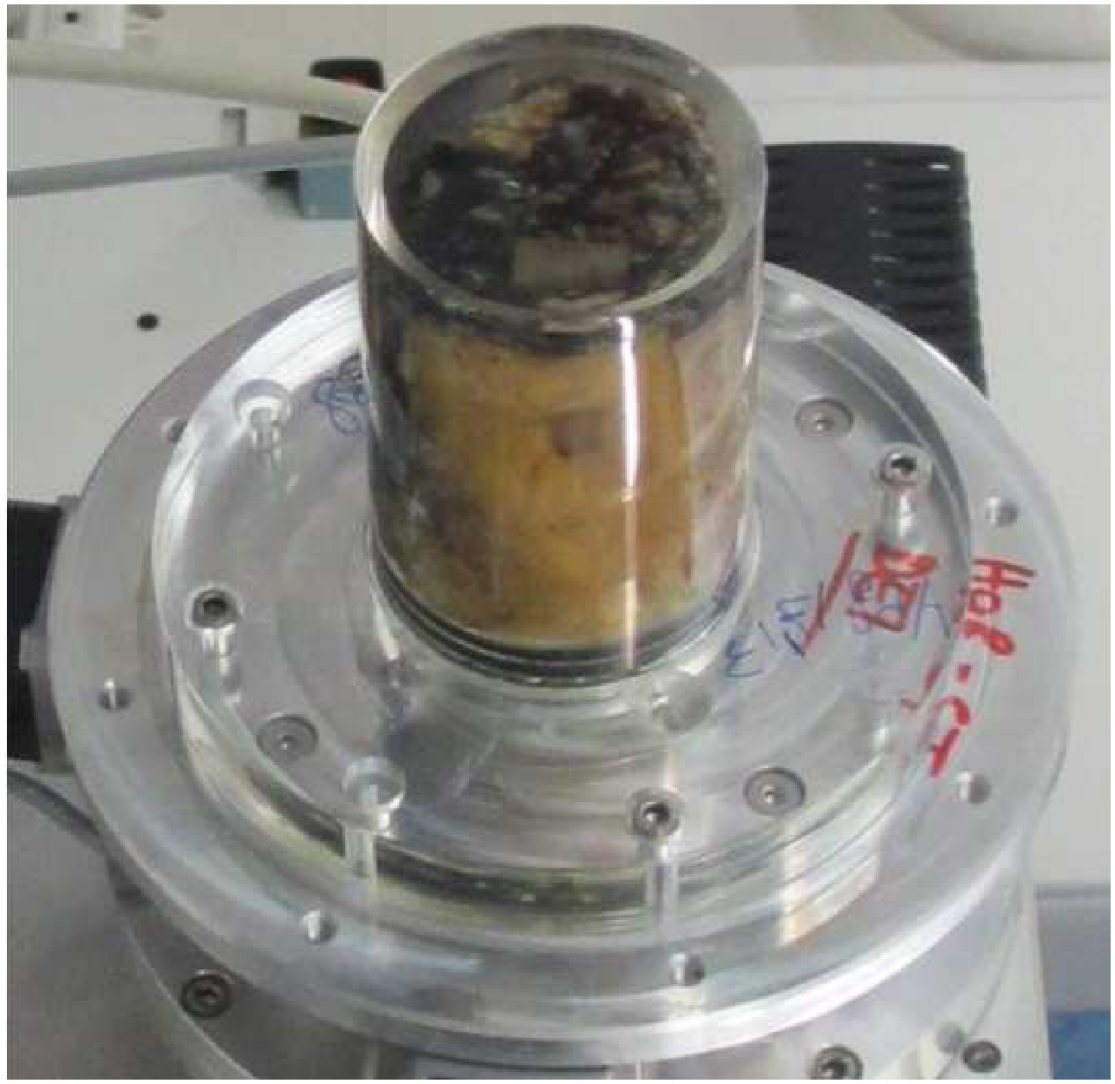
Sample-holder used for PC-CT imaging. Photograph of the sample-holder with the sample in it.
